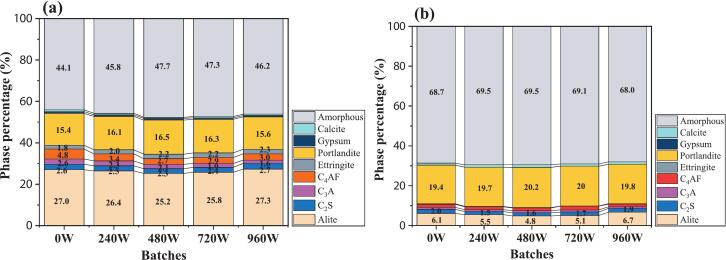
XRD patterns of the samples at different ultrasonic powers.
